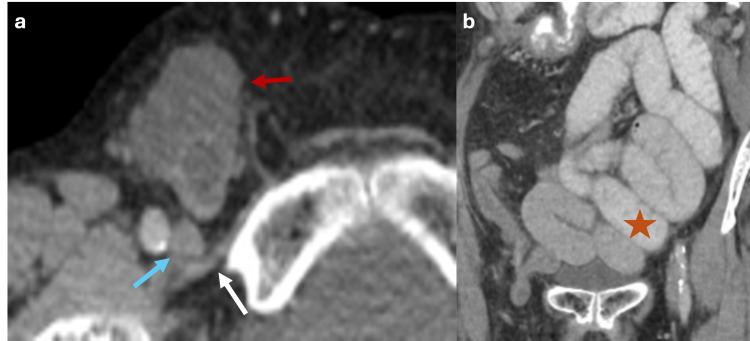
Axial and coronal CT scan of the abdomen with oral contrast showing a femoral hernia with small bowel obstruction (a, b) Axial and coronal CT scan of the abdomen with oral contrast displaying a femoral hernia with fluid and small bowel content (red arrow) located anterior to the pectineus muscle (white arrow) and medial to the femoral vein (blue arrow), leading to bowel obstruction (red star). The figure was created by the authors.
